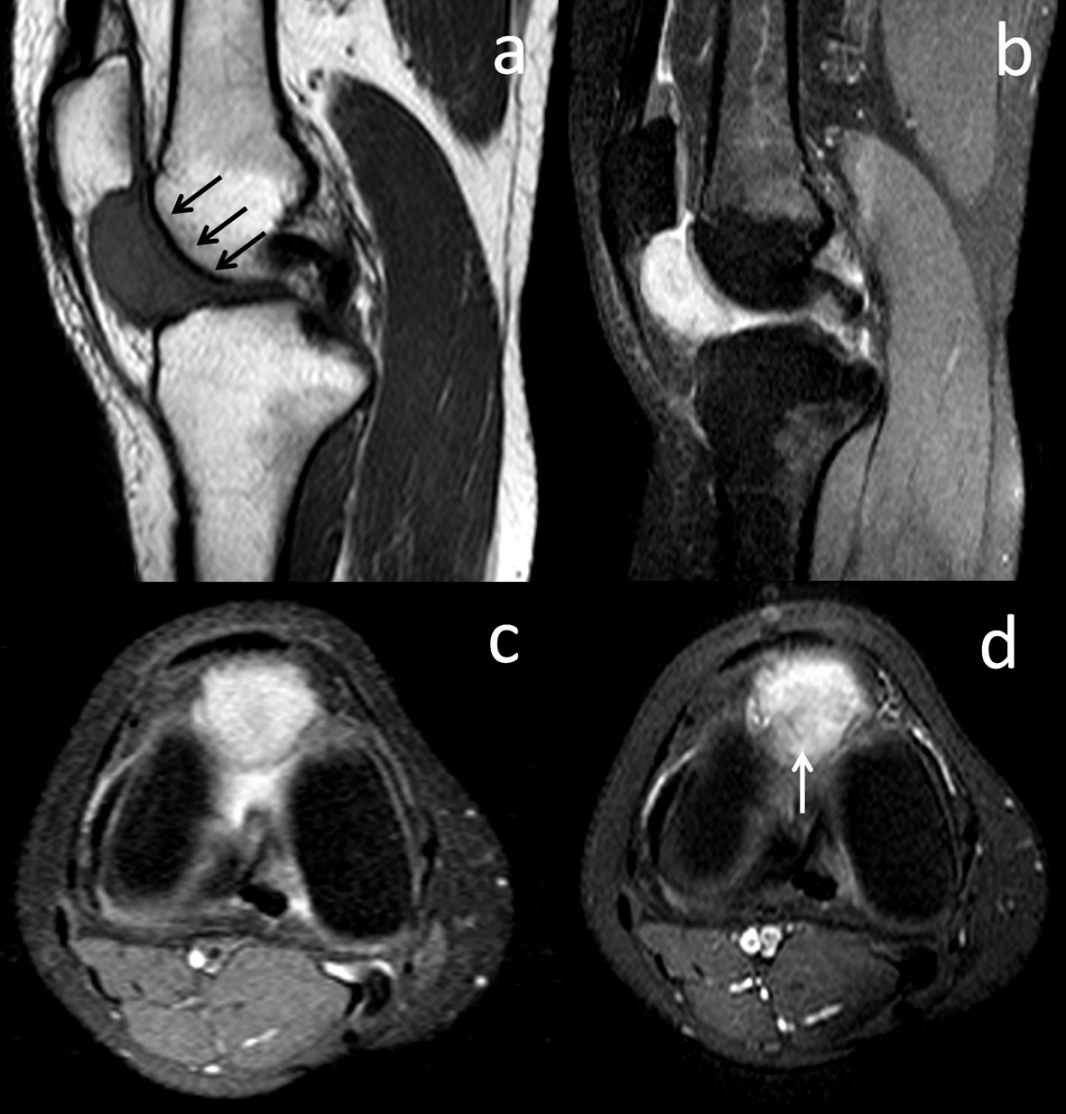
Preoperative MRI examination of the knee. MRI: magnetic resonance imaging
